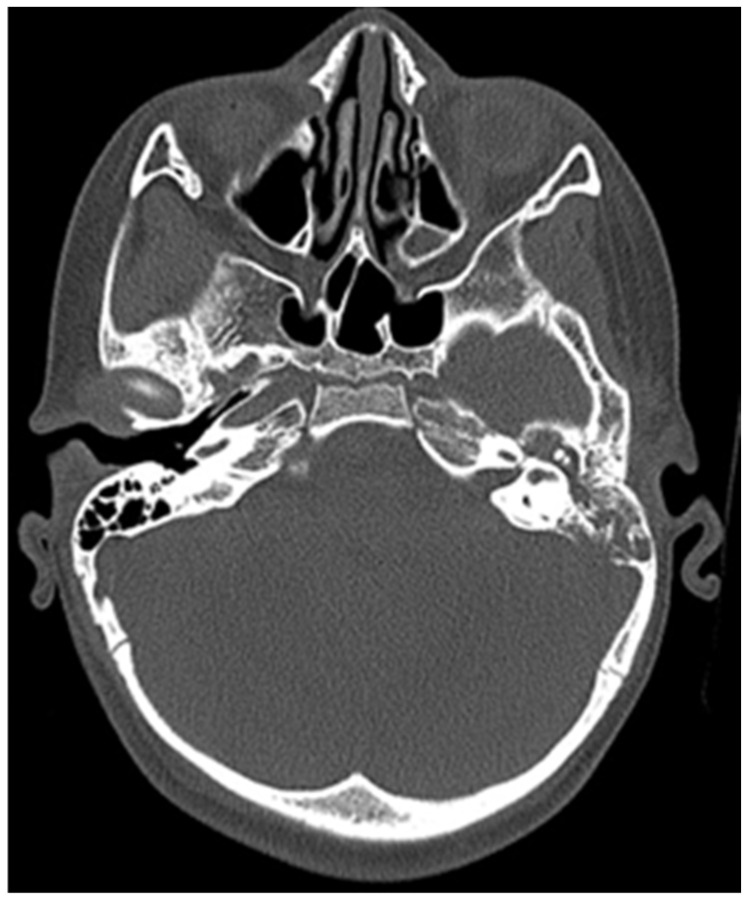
Initial CT of the patient.
